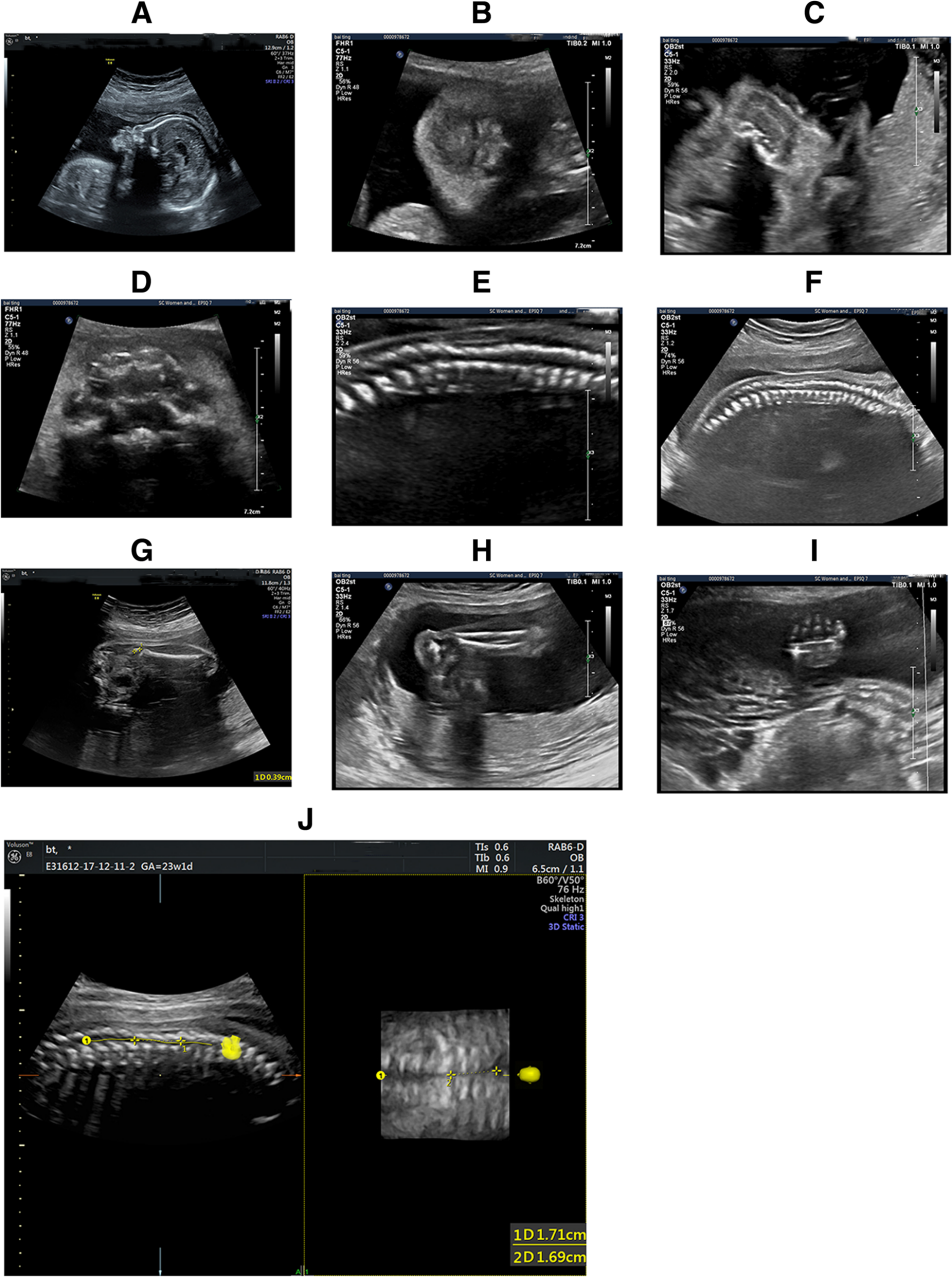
Antenatal ultrasonography features in our patient. a & b, Sagittal and coronal views of the fetal face showing facial flat, a depressed nose bridge, and collapsed nasal tip. c. Horizontal cross view of the fetal nasal tip show depressed, collapsed, and flat of the nasal tip. d. Transverse view of the upper alveolus shows that the upper alveolus is square. e & f. Sagittal view of the fetal spine shows changes in physiological curvature, T_9_-L_1_ spinal canal is narrow, the diameter of the thoracic spinal canal above the stenosis segment was about 4.1 mm, and that of the lumbar segment below the stenosis segment was about 4.0 mm. j. Three-dimensional ultrasound imaging of the fetal spine revealed T_9_-L_1_ spinal canal is narrow. g. Long axial view of the fetal femur show that early secondary ossification centers. h & i. There was no obvious abnormality of fetal ankle joint and hand ossification
